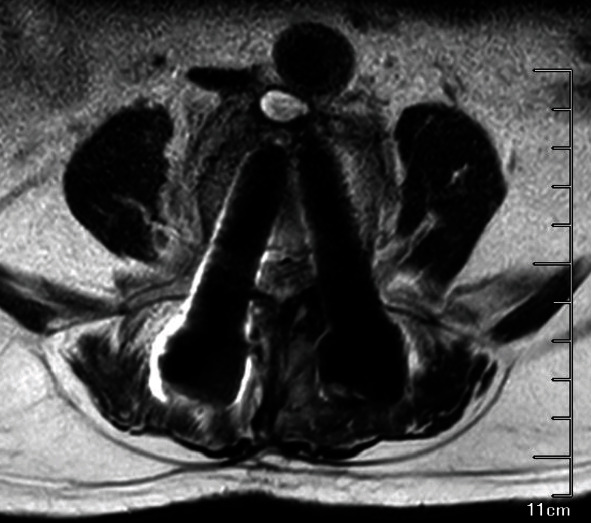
Preoperative MRI of the fourth and fifth lumbar spine.
